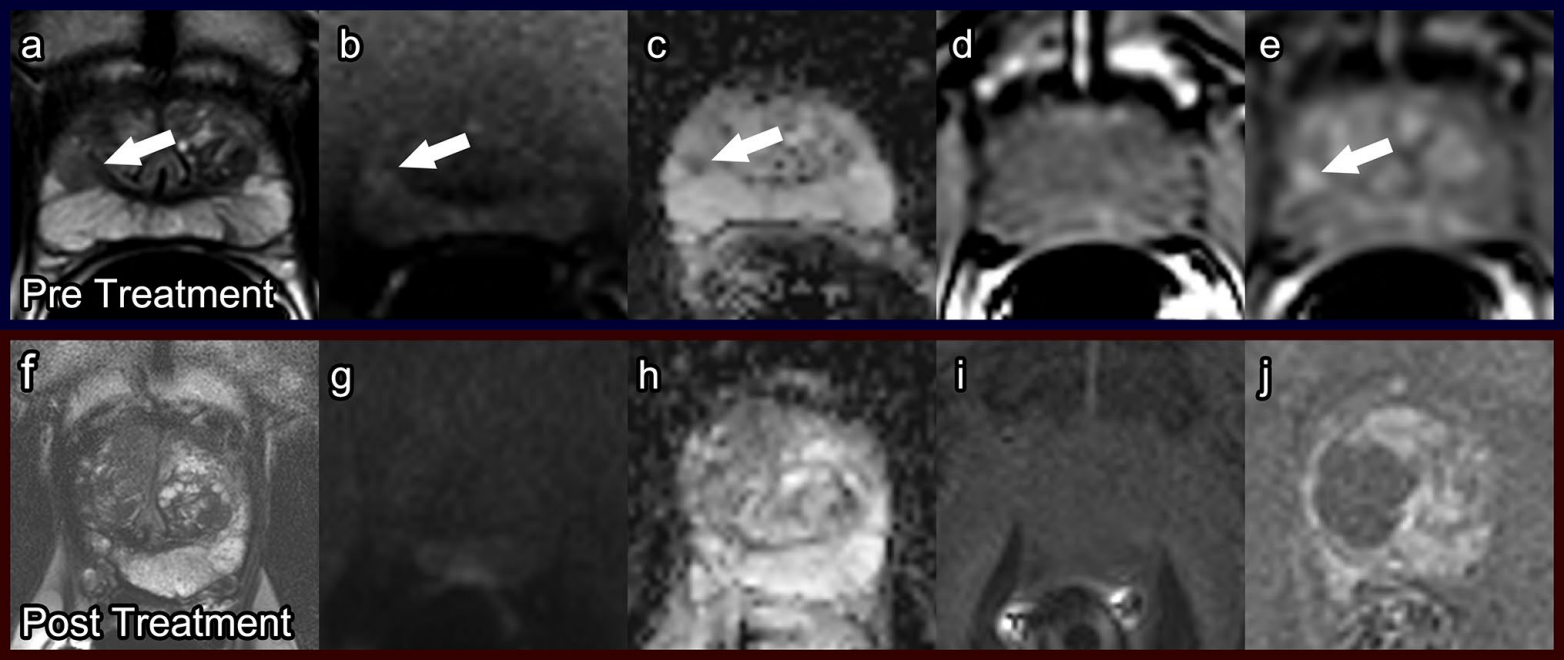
Focal laser ablation. Pre-treatment prostate MRI: Axial T2 (a), DWI (b), ADC (c), pre-contrast T1 (d), and post-contrast T1 (e) demonstrate a lesion at the right lateral mid-gland to apex peripheral zone (arrows). Post-focal laser ablation prostate MRI at 1 year: Axial T2 (f), DWI (g), ADC (h), pre-contrast T1 (i), and post-contrast T1 (j) demonstrate T2 hypointensity, atrophy, and retraction, with hypoenhancement in the ablated right lateral gland
